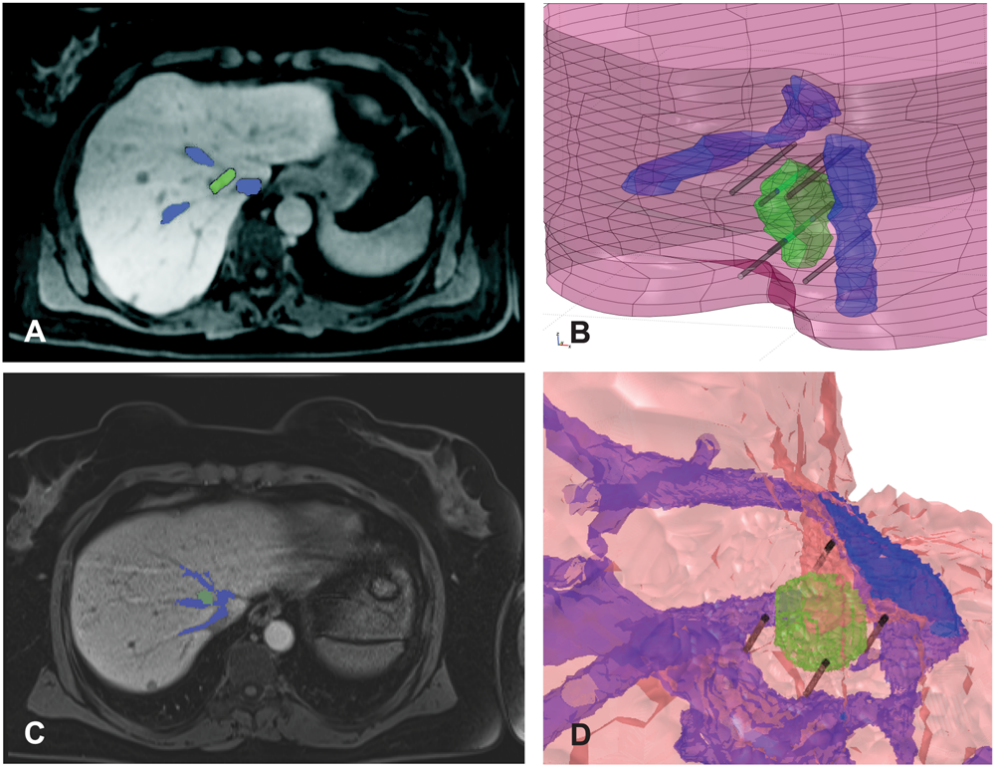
Real patient cases used in our study. A: MRI of the liver for first patient case. B: Reconstructed 3D model of the first case with inserted electrodes. C: MRI of the liver for second patient case. D: Reconstructed 3D model of the second case with inserted electrodes. In all images the structure colored green is the tumor, while the nearby major vessels are colored blue.
